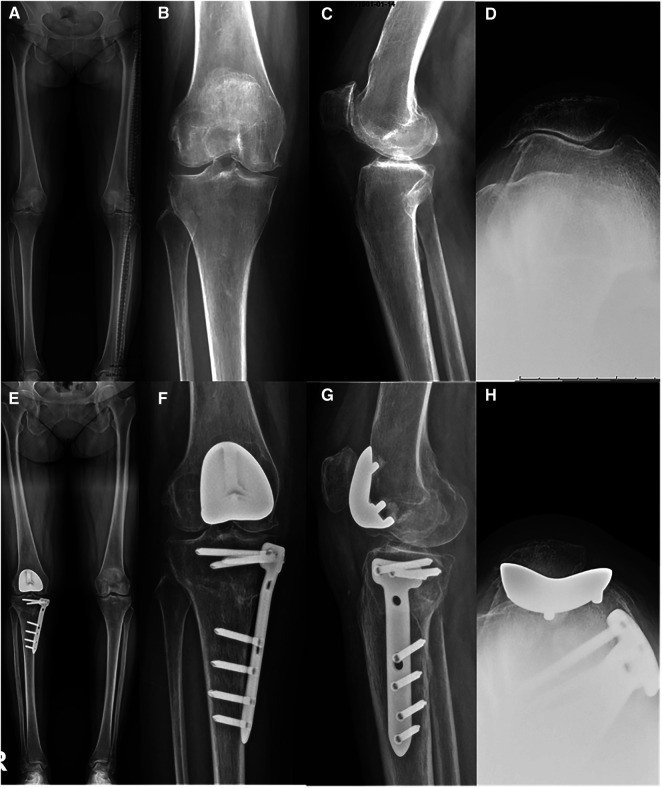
X-rays of a patient with the patellofemoral arthroplasty in combination with high tibial osteotomy preoperative (A–D) and postoperative at six months (E–H).
